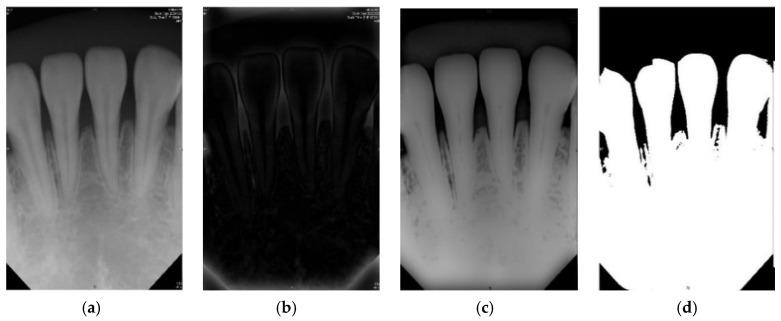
The preprocessed image results of this article. (a) Periapical film; (b) filtered result of the Gaussian high pass filter; (c) difference of (a) and (b); (d) result after the binarization.
